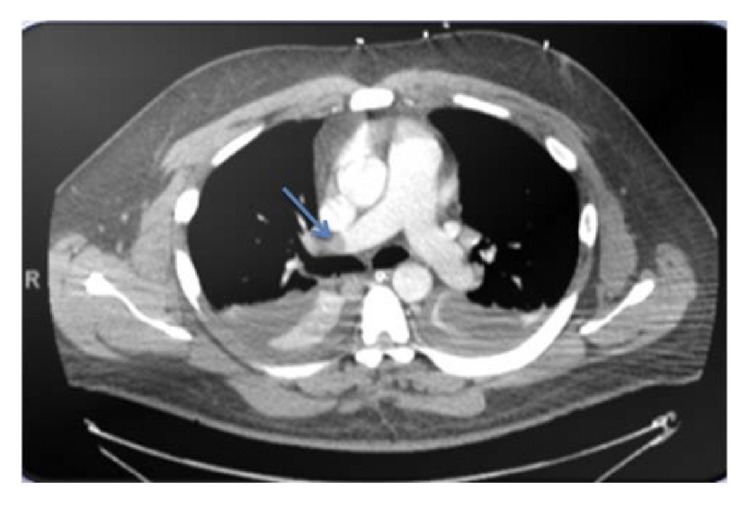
CT Chest demonstrating embolus in the right main pulmonary artery.
